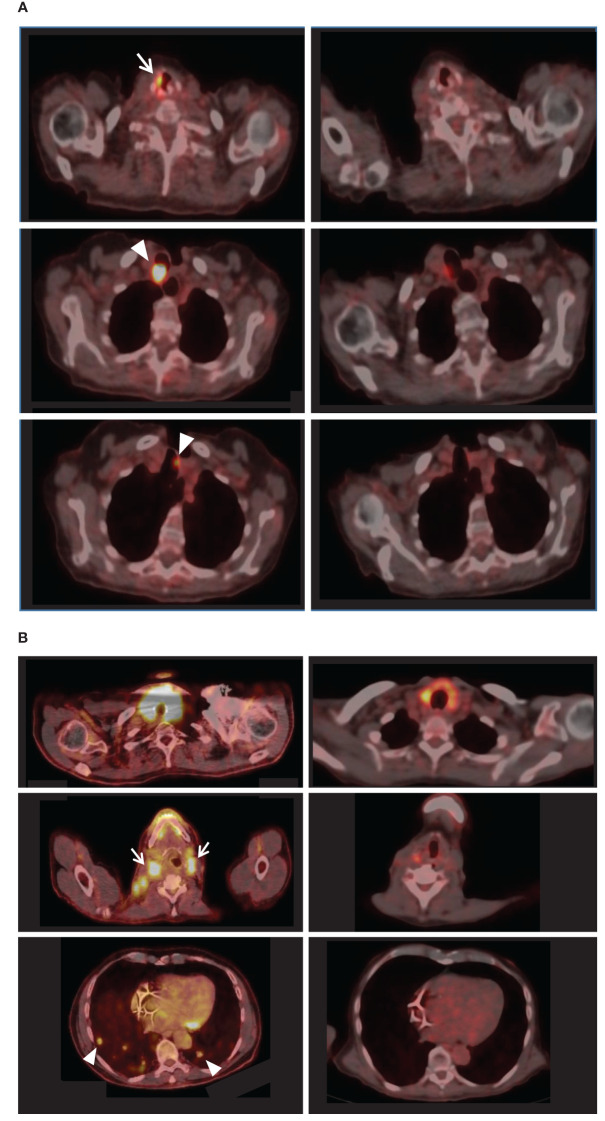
FDG-PET-CT results of the partial responders. For each patient, pre-intervention imaging results appear on the left, while comparable post-intervention sections appear on the right. (A)
